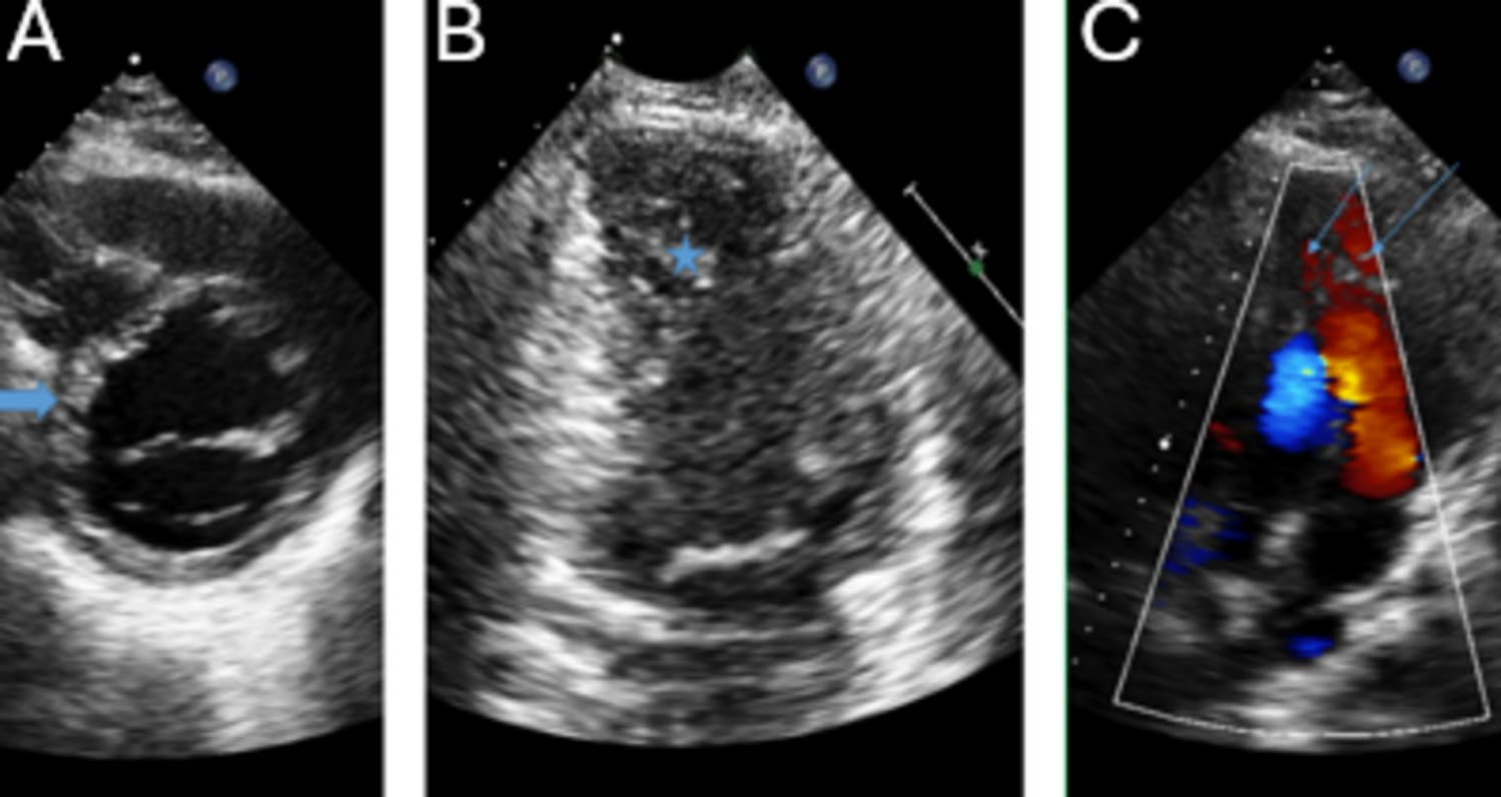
This is a patient with non compaction cardiomyopathy. There *thick arrow* demonstrates deep recesses (A). The *thin arrows* demonstrate color Doppler flow communicating into the left ventricular cavity (C). Prominent trabeculation is indicated by a *star* (B)
